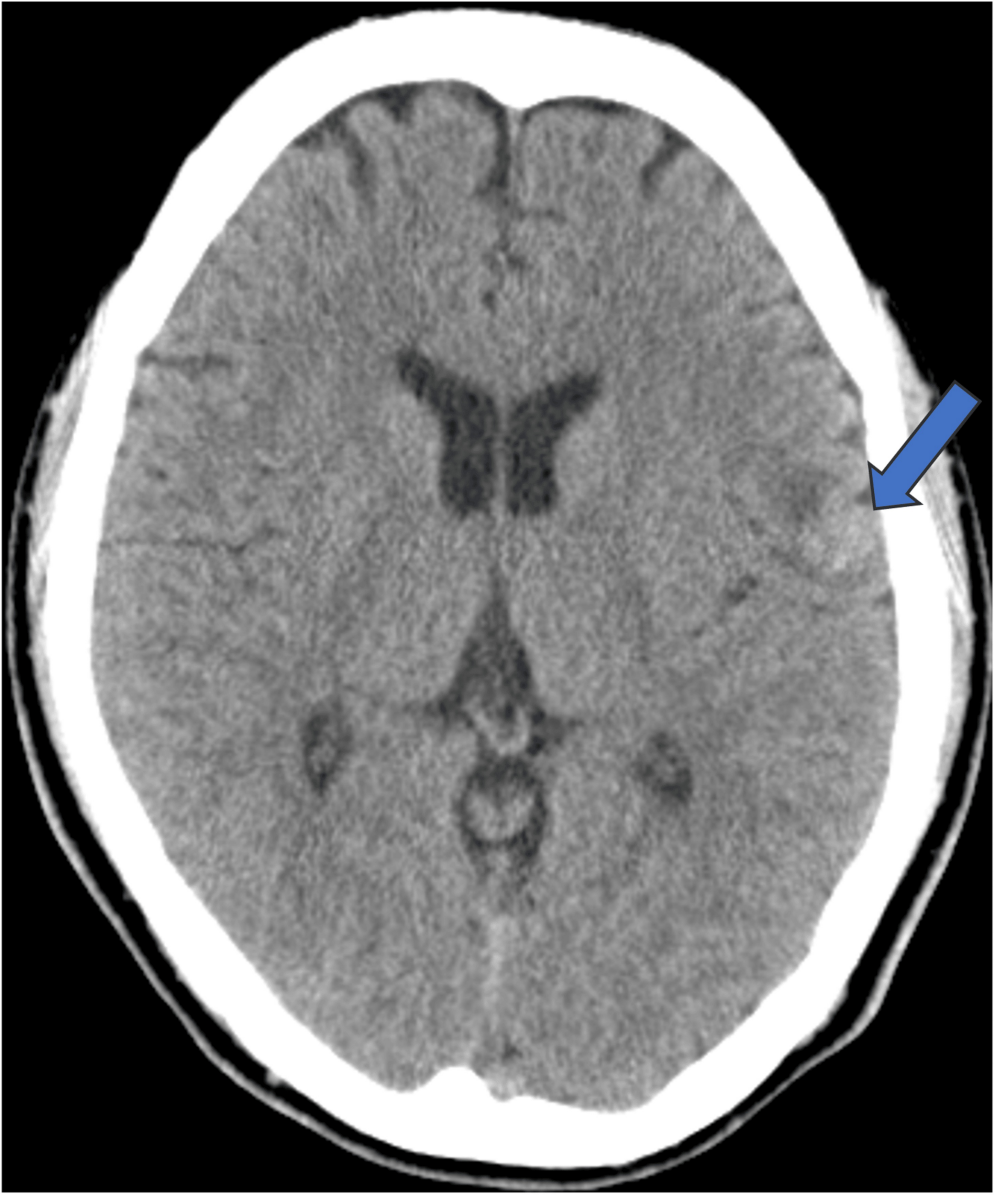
Plain CT shows the mass located in the left frontoparietal opercular subcortical white matter extending into the cortex (arrow). The mass shows isoattenuation compared to the normal gray matter, with surrounding white matter displaying low-attenuation areas suggestive of edematous changes. No obvious calcification or hemorrhage is observed.
